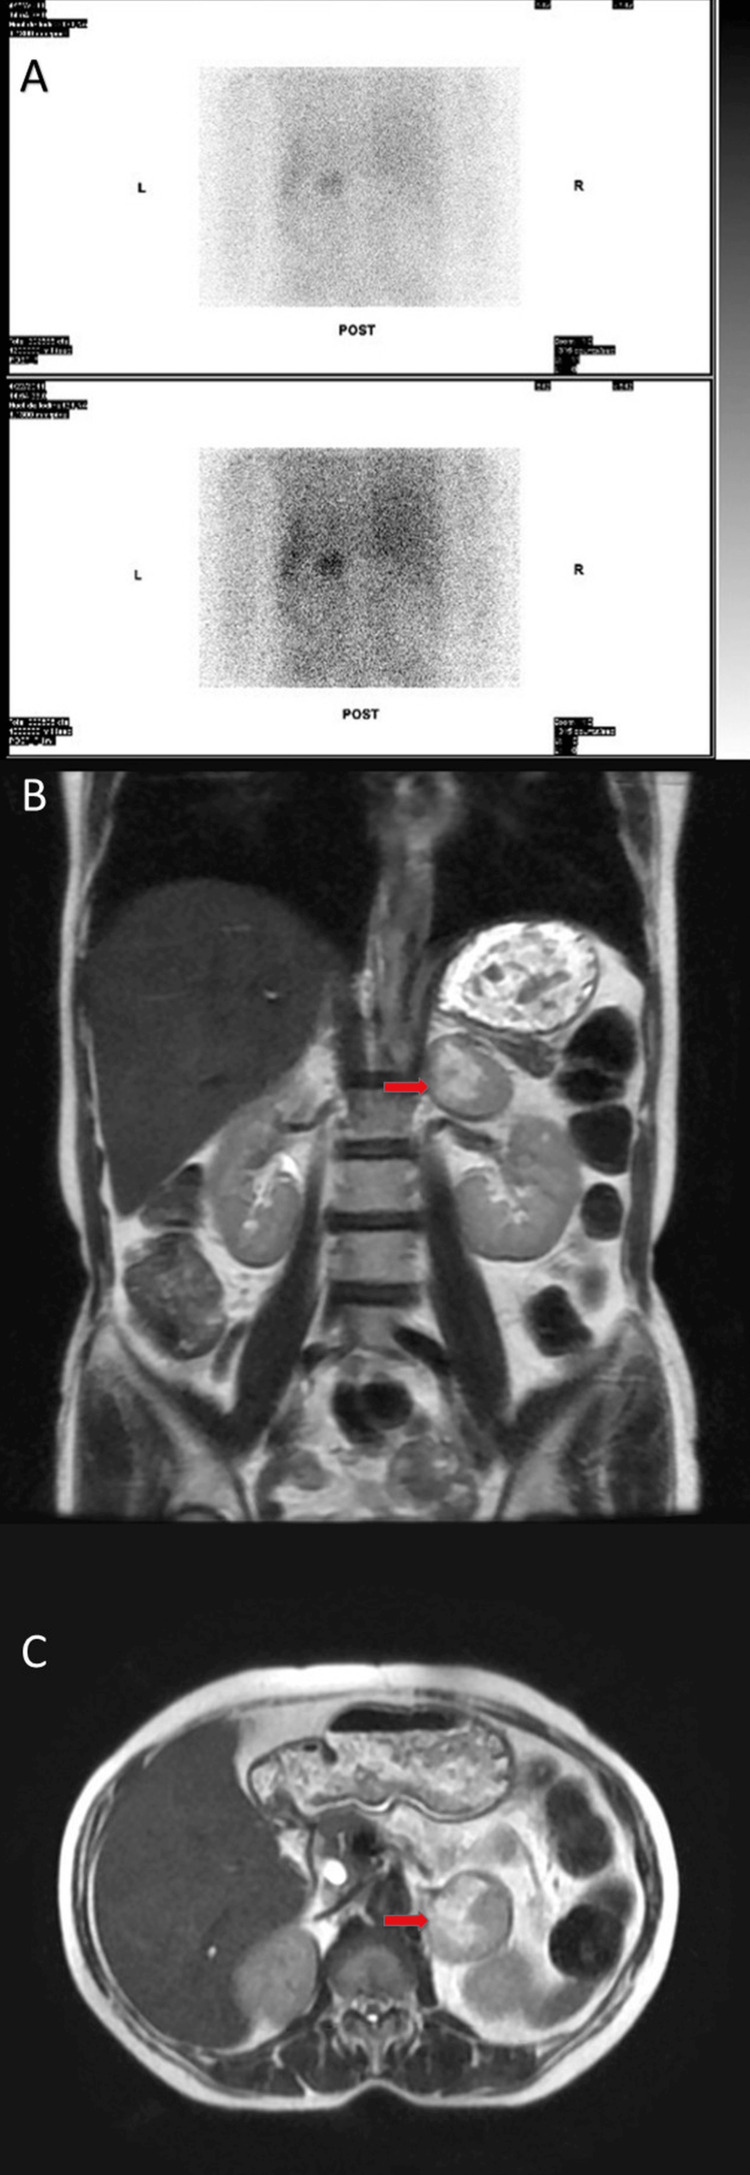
Imaging Findings Consistent With Left Adrenal Pheochromocytoma (A) Iodine-131 metaiodobenzylguanidine (^131^I-MIBG) scintigraphy showing focal radiotracer uptake in the left adrenal region, consistent with a catecholamine-secreting tumor. (B) Coronal T2-weighted MRI demonstrating a well-circumscribed, hyperintense mass in the left adrenal gland measuring approximately 4.4 cm in diameter (red arrow). (C) Axial T2-weighted MRI confirming the adrenal lesion with high signal intensity and smooth, ovoid morphology (red arrow).
